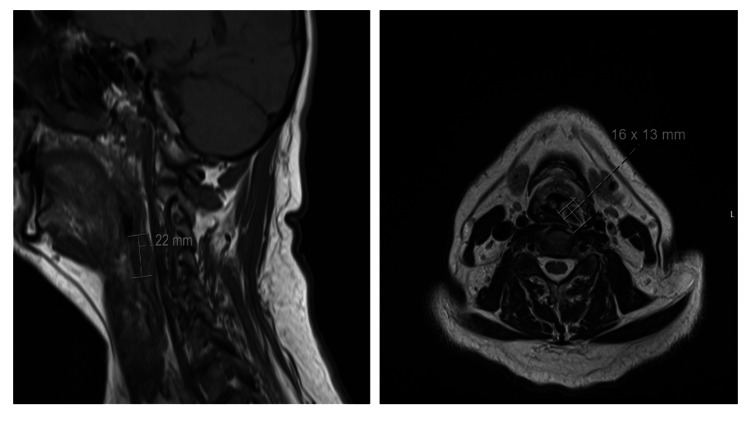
MRI of the C-spine revealed a 1.6 x 1.3 x 2.2 cm submucosal mass in the left posterior lateral hypopharynx (lateral and cross-section images).
